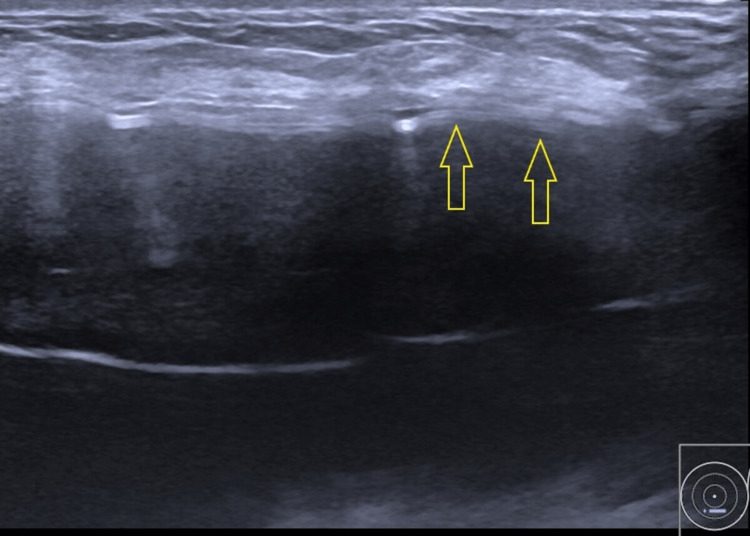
Left breast ultrasound showing a stepladder sign (yellow arrows), indicating an intracapsular implant rupture.
